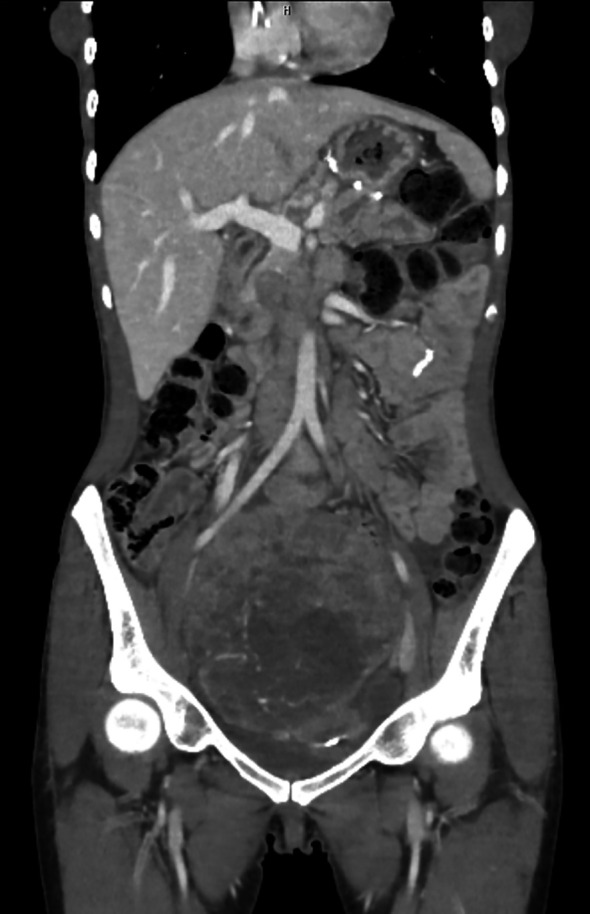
Computed tomography scan of the patient. An abdominal computed tomography (CT) scan showed a 135x120 mm, well-circumscribed, heterogeneous mass filling the pelvic region. It displayed intense heterogeneous contrast enhancement with central hypodense areas suggestive of necrosis and compressed both bilateral external and internal iliac veins. No liver metastasis or peritoneal implants were observed.
